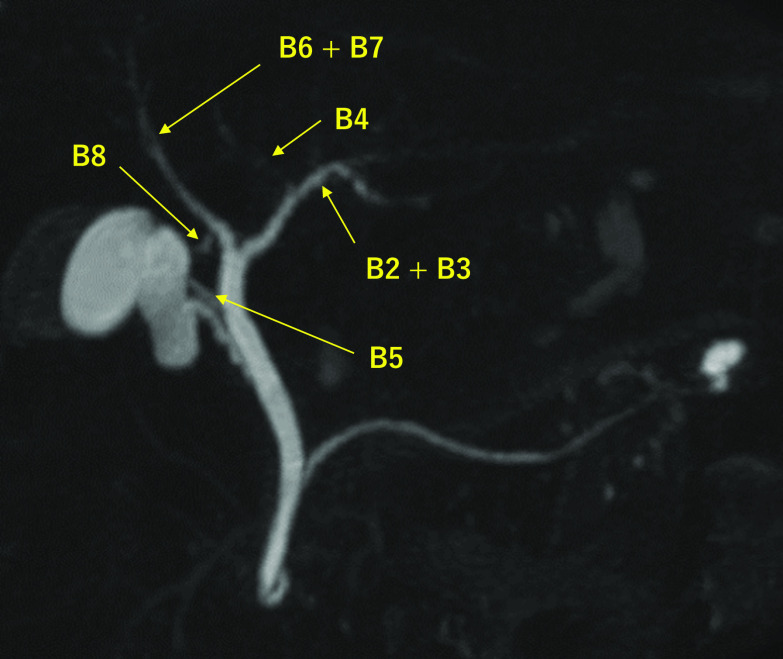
A 69-year-old woman presenting with abdominal pain and gallbladder stone in ultrasound examination. Magnetic resonance cholangiopancreatography showing an aberrant biliary duct of liver segment 5 branching directly off the common hepatic duct
